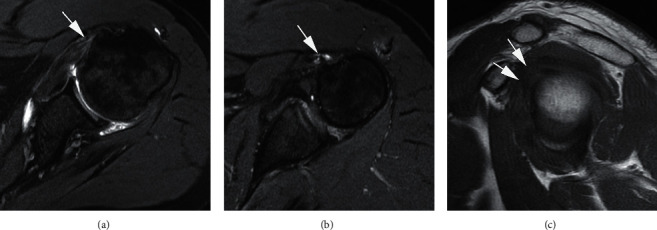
A 32-year-old man with left shoulder pain after dislocation. Axial T2-weighted FS MRI (a) and (b) show an articular surface partial tear of the subscapularis tendon (arrow in a) with tearing and medial dislocation of the long head of the biceps tendon (arrow in b). Sagittal oblique T1-weighted MRI (c) displays loss of normal fat within the rotator interval (arrows).
